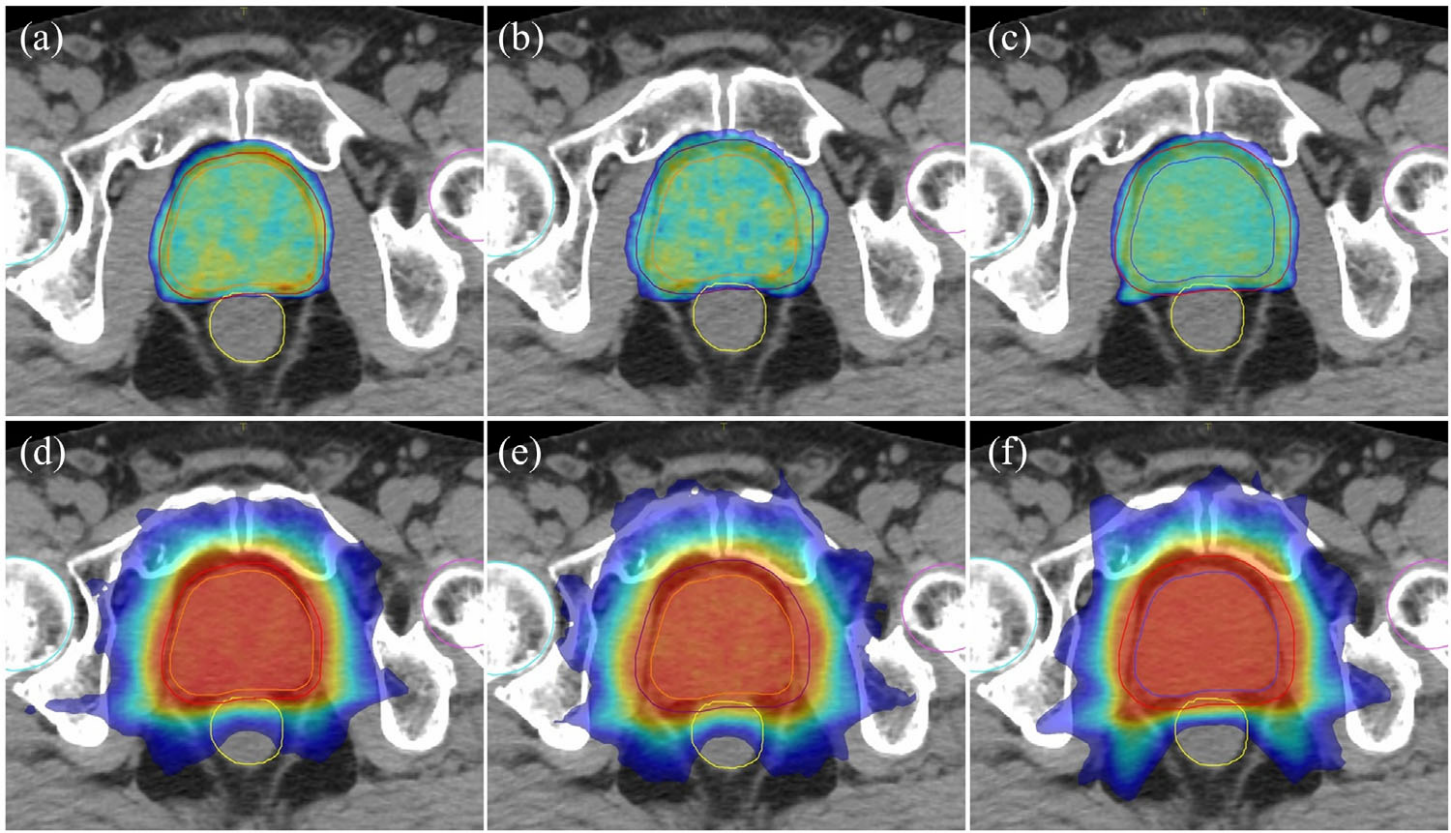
Representative axial CT slices showing 95% of prescribed dose distributions (green area) for the (a) MR‐Linac plan with reduced margin (MRL‐R), (b) MR‐Linac plan with normal margin (MRL‐N), and (c) intensity‐modulated radiotherapy plan and 50% of prescribed dose distributions (blue area) for the (d–f) same consecutive plans (red area represents 95% isodose area).
